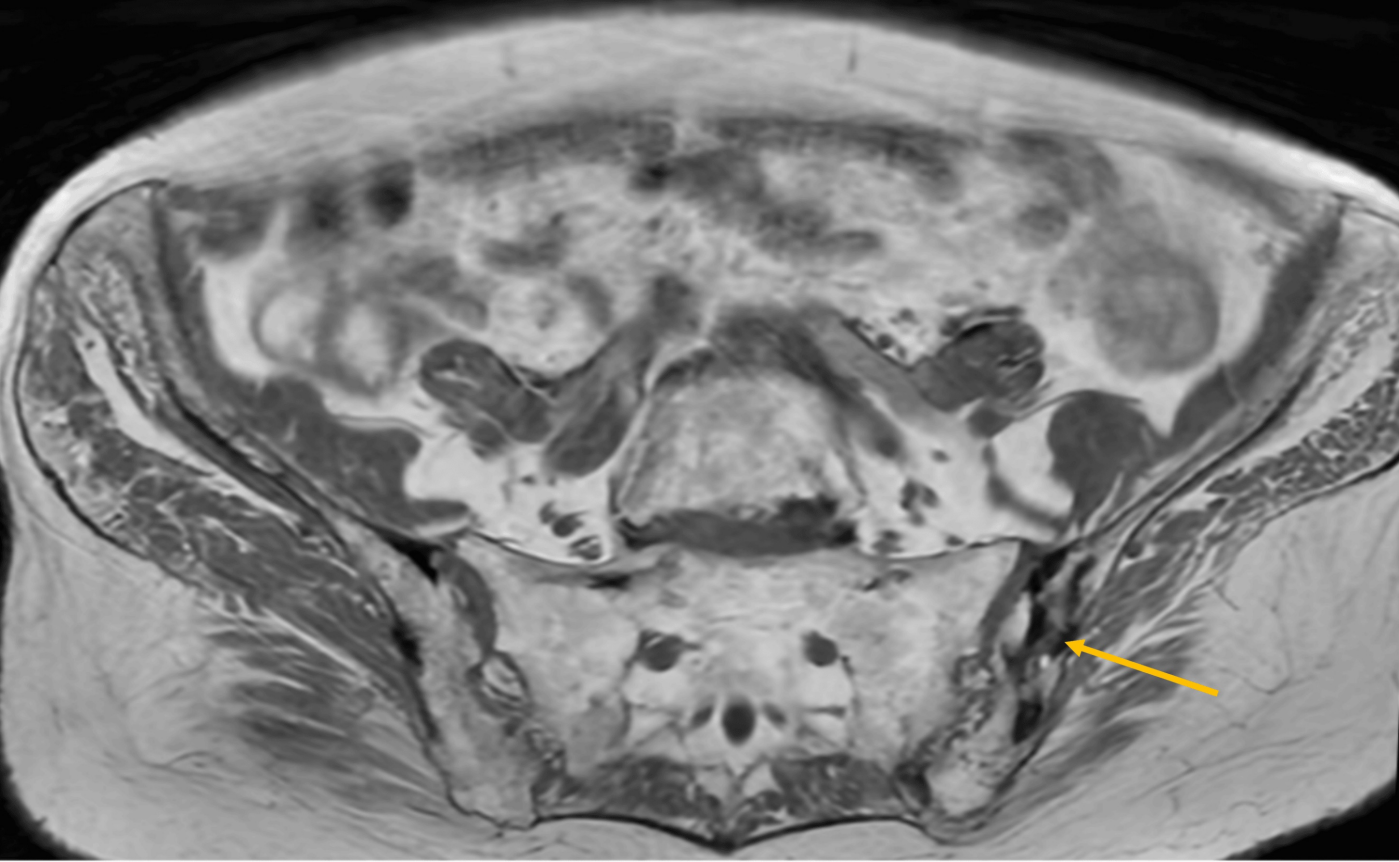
Paget’s disease of the left iliac bone. Axial T1-weighted image shows heterogeneous low signal in the left iliac bone (orange arrow), compatible with the patient’s known Paget’s disease.
